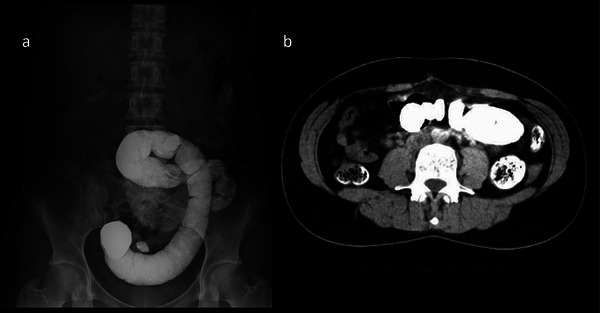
Abdominal X‐ray and contrast‐enhanced computed tomography revealed a large amount of retained barium in the sigmoid colon without evidence of free air. (a) Abdominal X‐ray, (b) Abdominal computed tomography.
